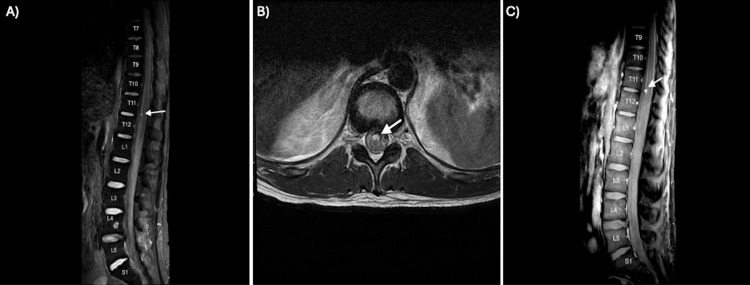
MRI of the thoracolumbar spine with STIR sequence (A), axial T2-weighted sequence (B), and post-contrast T1-weighted sequence (C) showing diffuse abnormal signal through subarachnoid space with 6mm enhancing lesion noted anteriorly in the thecal sac at the T11-T12 level (noted as an arrow). MRI, magnetic resonance imaging; STIR, short T1-inversion recovery
